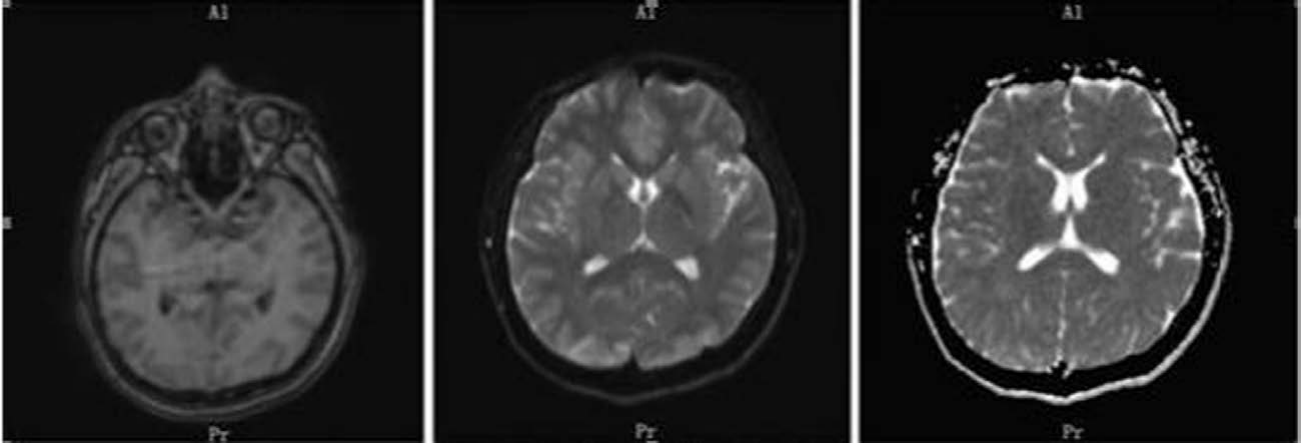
MRI scan of head revealing frontal and occipital lobe abnormalities with suspected posterior reversible encephalopathy syndrome characterized by reversible brain edema. MRI = magnetic resonance imaging.
